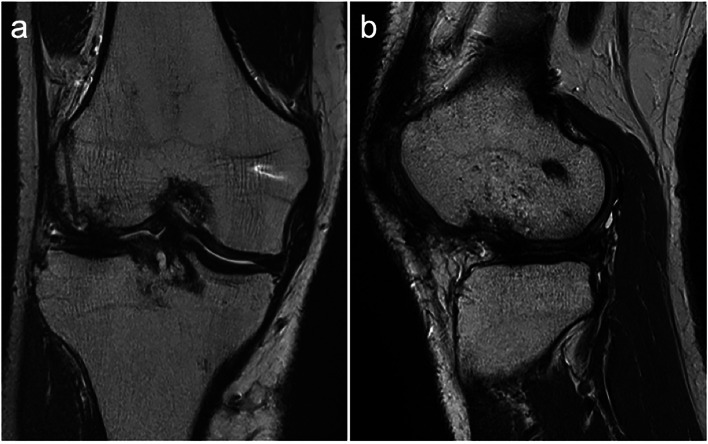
Magnetic resonance imaging (MRI) evaluation at 4 years after surgery. a Coronal T2-weighted, b Sagittal T2-weighted MRI images of the right knee demonstrating integration of graft tissue to border zone and subchondral bone
